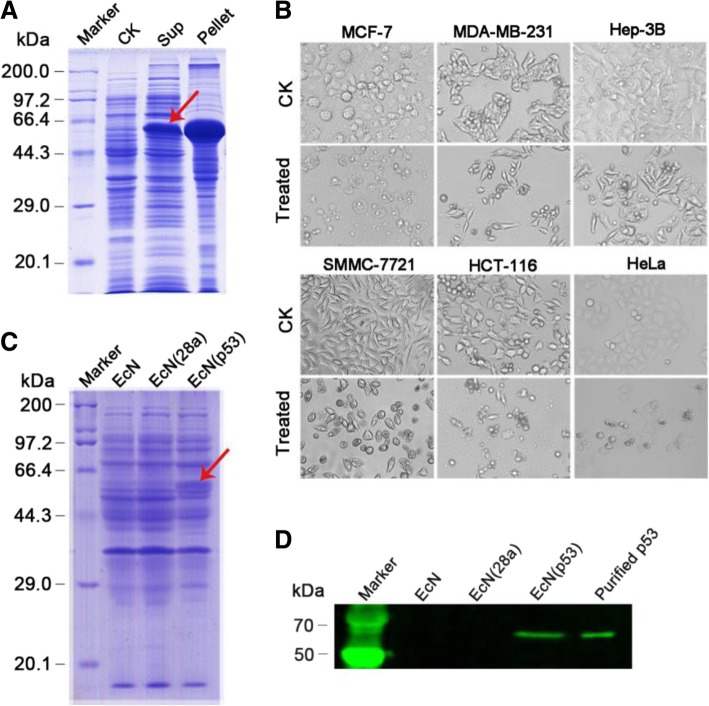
Efficient expression of p53 protein in *E. coli* BL21 (DE3) and probiotic EcN. a p53 expression levels in cell lysates (Pellet) or supernatants (Sup) were detected by subjecting *E. coli* BL21 (DE3) bearing *p53*-gene-expressing plasmid or empty plasmid (CK) to SDS-PAGE after IPTG induction and ultrasonication. b p53 protein could inhibit the proliferation of various types of cancer cells. PBS (CK) and 40 μg/mL recombinant p53 protein were coincubated with MCF-7, MDA-MB-231, Hep-3B, SMMC-7721, HCT-116, HeLa tumor cells, and CCK-8 solution was used to calculate the inhibitory effect of the recombinant protein on tumor cells. SDS-PAGE (c) and Western blot analysis (d) of p53 expression in EcN, EcN (28a), and EcN (p53)
